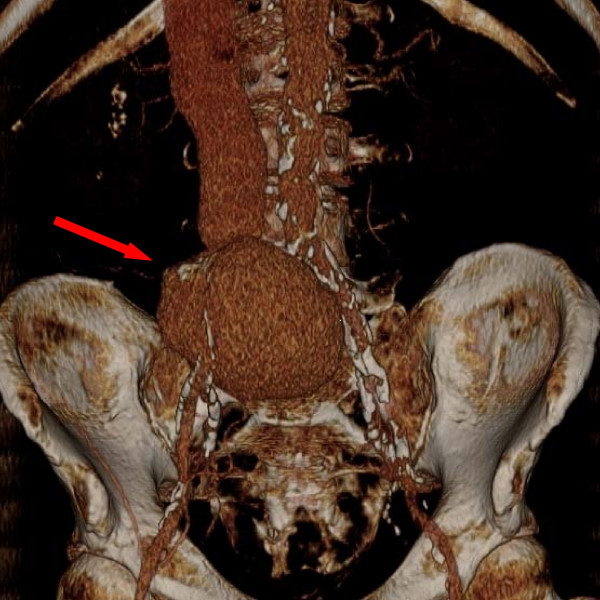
Preoperative CT. Preoperative contrast-enhanced CT 3-D reconstruction with arrow demonstrating the 8 cm ilio-iliac AV fistula originating from the proximal right CIA.
